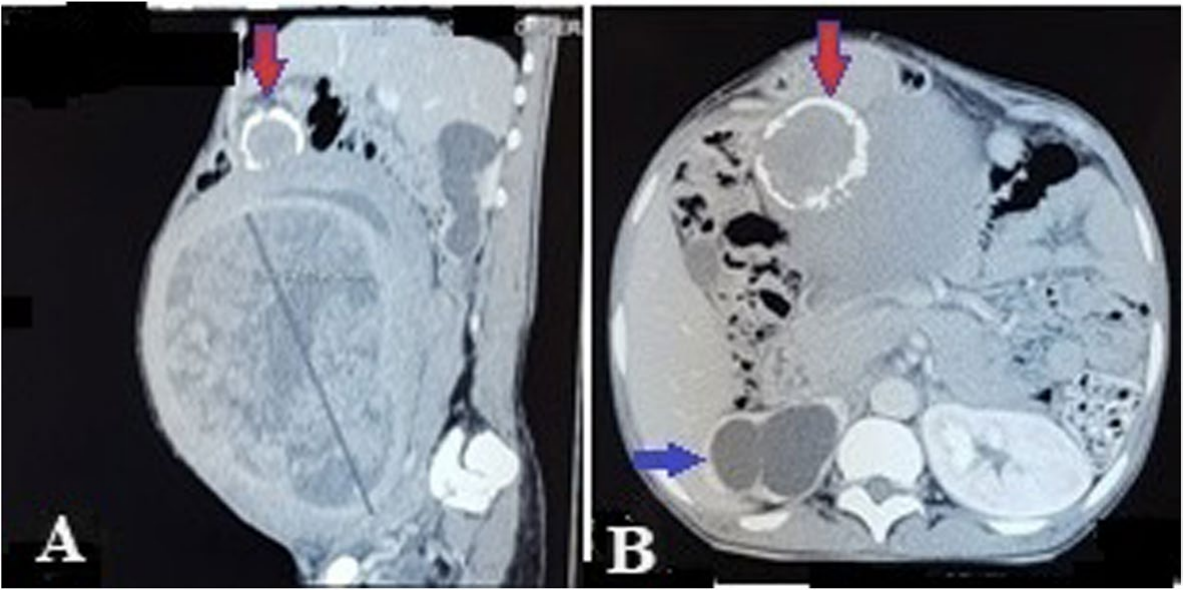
CT abdomino pelvis injected with a large mass abdominal cavity: frontal section (A) Poly nodular fluid tissue mixed tumor process occupying virtually the entire abdominal cavity, with thick and even wall taking contrast; a calcified tissue nodule (red arrow). The measurements were 210,64 × 172,69 × 229 mm (B) axial section: The lesion exerts a mass effect on the urinary tract and the bladder giving a right hydronephrosis uretero, pyelic diameter 18, 89 mm (blue arrow)
